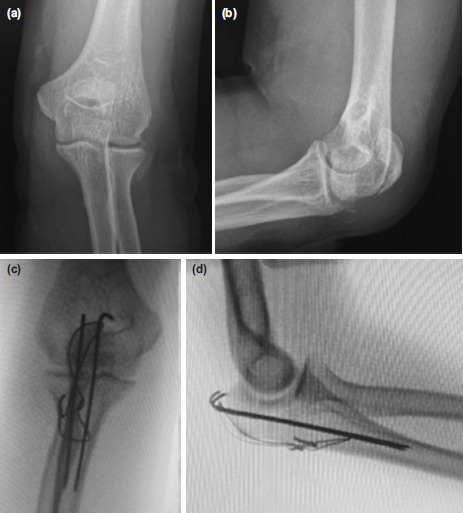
Case radiographs. (a) Pre-operative elbow AP and (b) Lateral views. (c) Final elbow AP and (d) Lateral views.
